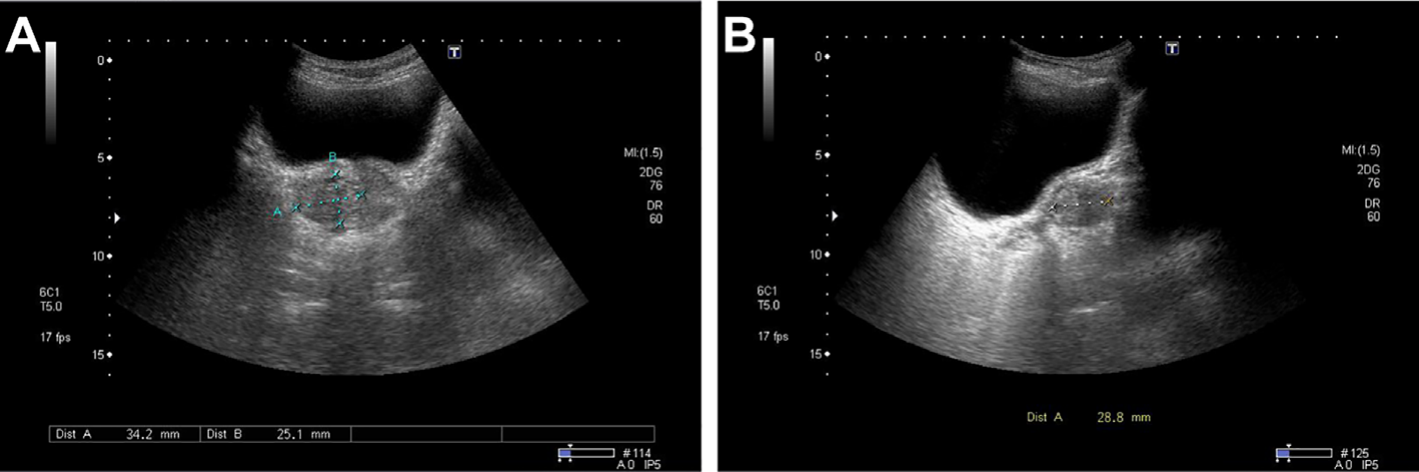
Ultrasound detected a non-uniform hypoechoic nodule, about 34 mm × 25 mm × 29 mm in size, in the right lateral lobe of the prostate with the obscure boundary. No marked color flow signal was observed within the lesion upon Color Doppler flow imaging. (A) Transverse position. (B) Sagittal position.
